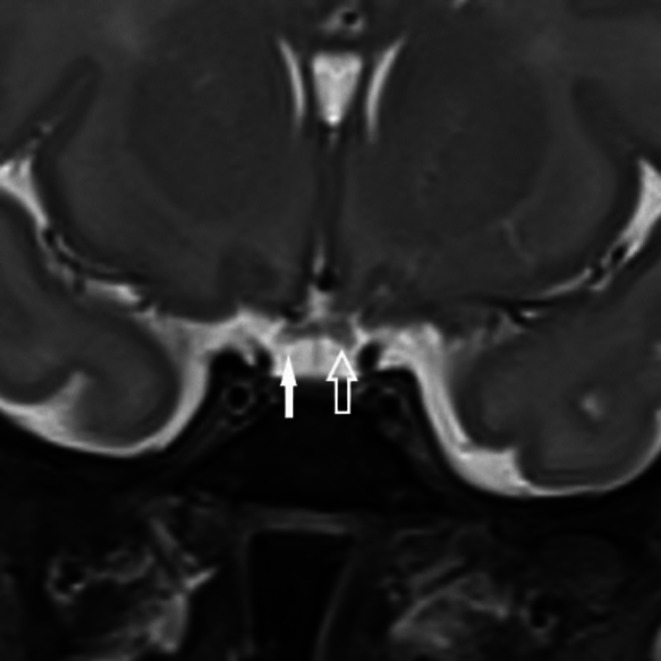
Coronal T2‐weighted fine section MR image shows the right aspect of the optic chiasm (solid arrow) to be slightly smaller than the left (open arrow).
